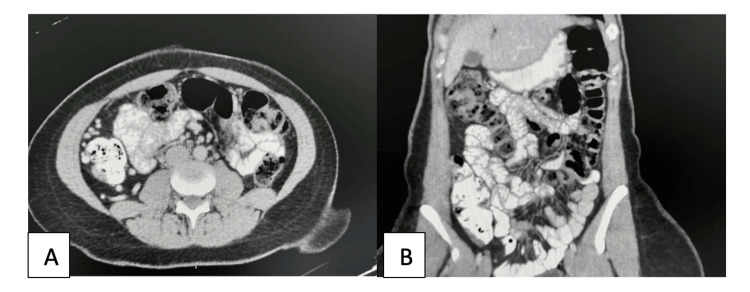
Contrast-enhanced CT scan of the abdomen (A) Axial contrast-enhanced CT image demonstrating eccentric thickening of the inferior cecal wall (B) Coronal view showing focal mural thickening at the ileocecal region without evidence of bowel obstruction or active intussusception
